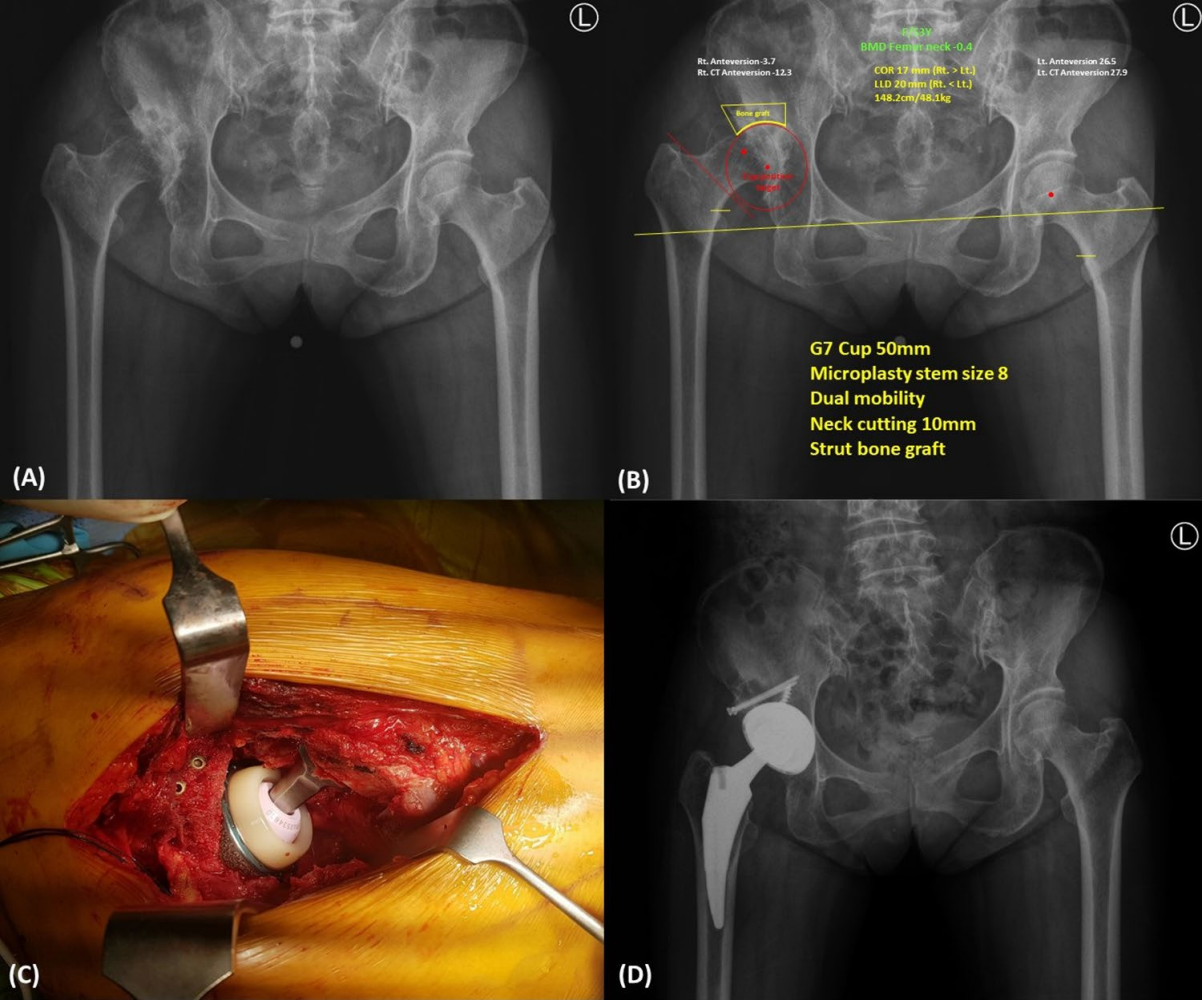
Sample images of DM THA in hip dysplasia patient. (A) Preoperative radiograph, (B) Templating using PACS, (C) Intraoperative photo, (D) Postoperative radiograph.
